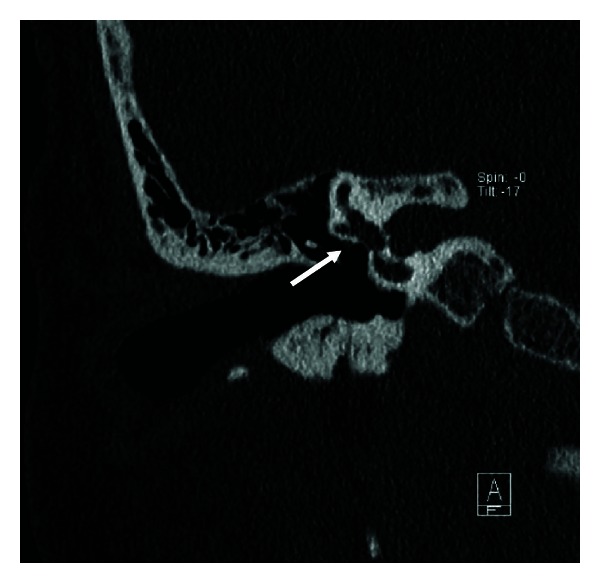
Facial nerve dehiscence. Coronal CT image of right temporal bone demonstrates dehiscence of right CN VII tympanic segment at the level of oval window (arrow).
